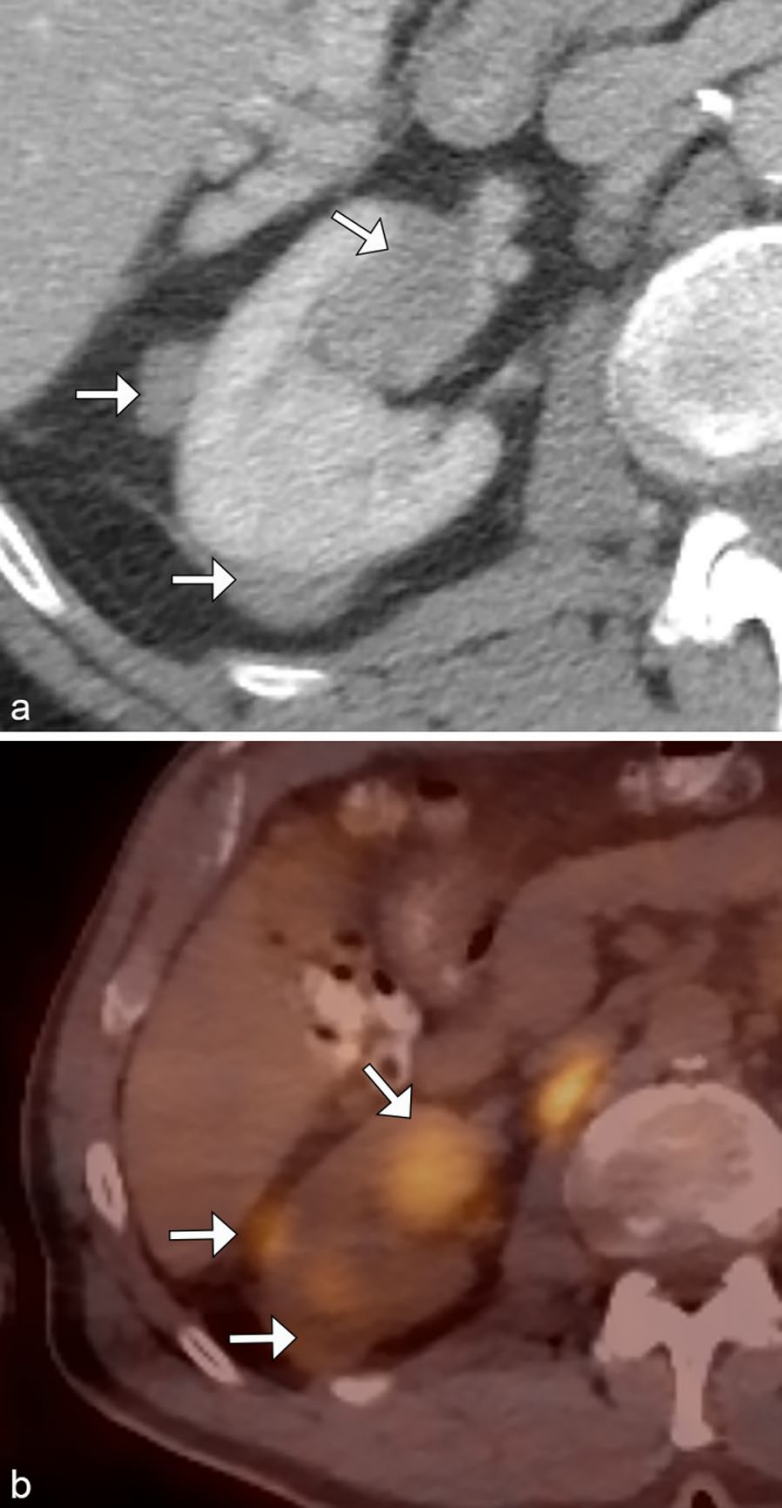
Multiple renal masses of renal lymphoma*. Axial contrast CT (a) demonstrates small, hypoenhancing masses (arrows) in the right kidney. Axial fused PET/CT image (b) demonstrates F18-FDG avidity of these masses (arrows) consistent with lymphoma in this patient with known diffuse large b-cell lymphoma
